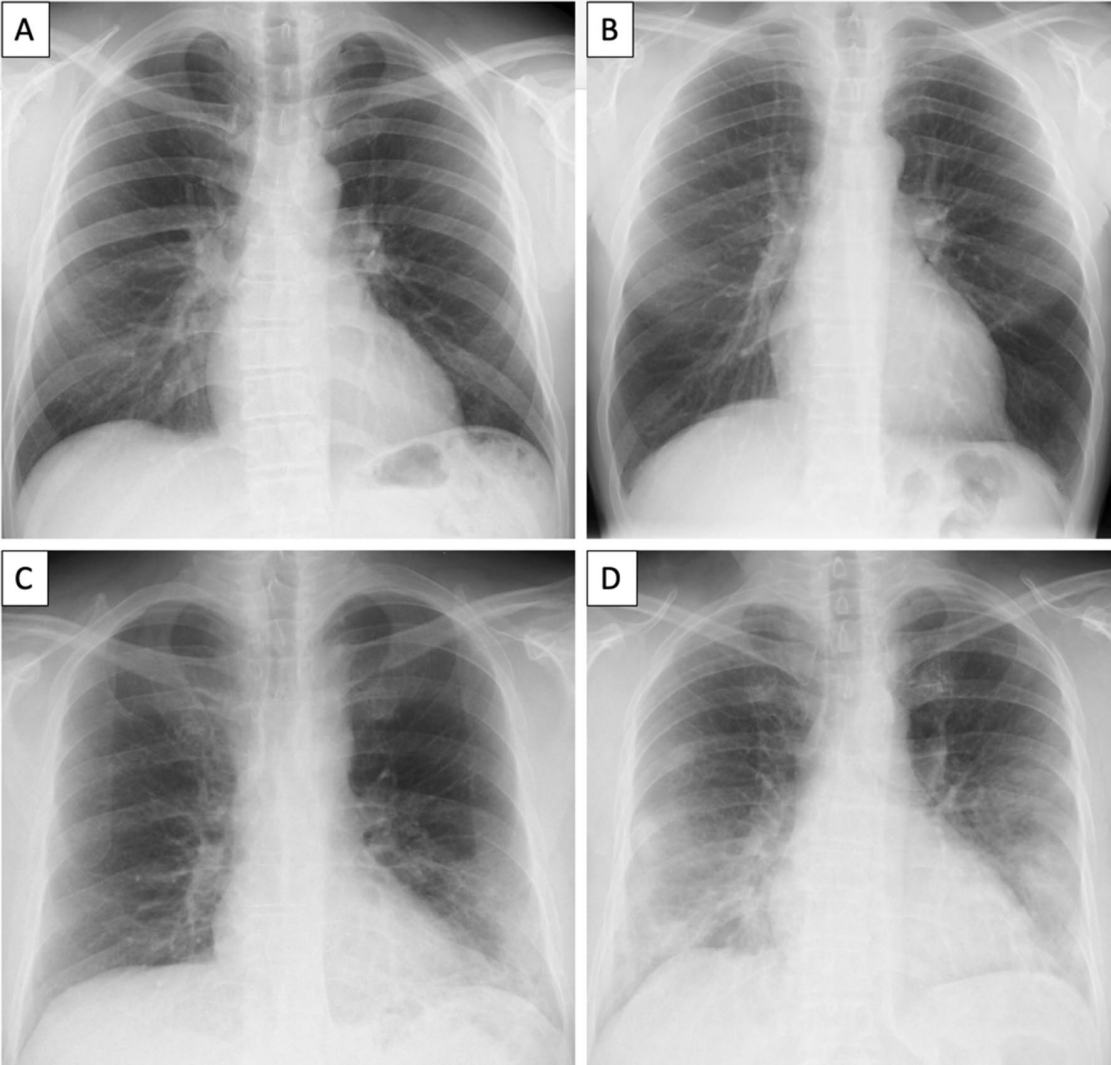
Baseline chest X-ray finding in COVID-19 patients associated with clinical and laboratory data. a 47-year-old man, without comorbidities, presented with fever, cough, rhinitis and conjunctivitis for 3 days. Laboratory test: Lymphocytes 1.31 (10^9^/L), CRP = 8.3 mg/L and LDH = 330 UI/L. The chest X-ray resulted negative. b 46-year-old man, without comorbidities, presented with fever, cough, and Pharyngodynia for 1 days. Laboratory test: Lymphocytes 0.8 (10^9^/L), CRP = 3 mg/L and LDH = 346 UI/L. The chest X-ray resulted negative. c 58-year-old man, without comorbidities, presented with fever and cough for 7 days. Laboratory test: Lymphocytes 0.67 (10^9^/L), CRP = 141.9 mg/L and LDH = 711 UI/L. The chest X-ray resulted positive with peripheral airspace opacification in the left lower lobe. D: 55-year-old woman, without comorbidities, presented with fever and cough for 7 days. Laboratory test: Lymphocytes 1.13 (10^9^/L), CRP = 60.9 mg/L and LDH = 784 UI/L. The chest X-ray resulted positive with neither peripheral nor perihilar airspace opacification with middle and lower zone involvement
